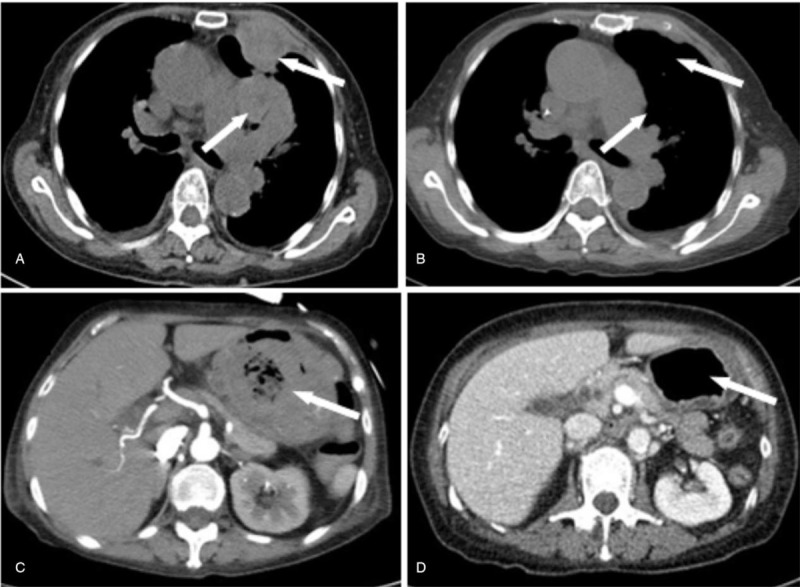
Chest and abdominal CT scan showing multiple pulmonary, pleural, and gastric lesions. A and B, in Case 1 upon the last progression, the largest one with a diameter of 7.7 cm, which disappeared. C and D, After lenalidomide plus chidamide therapy. CT = computed tomography.
